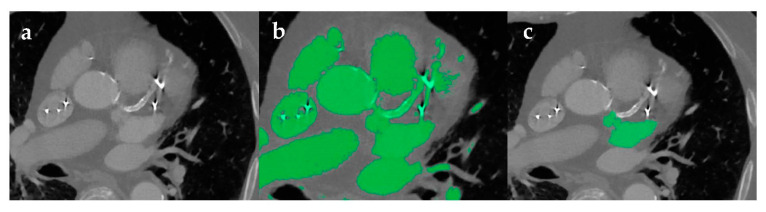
Image segmentation process with artifacts. (a) Axial contrast enhanced CT of the heart demonstrates bright artifacts as a result of a metal implant and arterial calcification. (b) These artifacts diminish the efficacy of automatic segmentation via thresholding. (c) Manual segmentation was used to isolate the area of interest.
